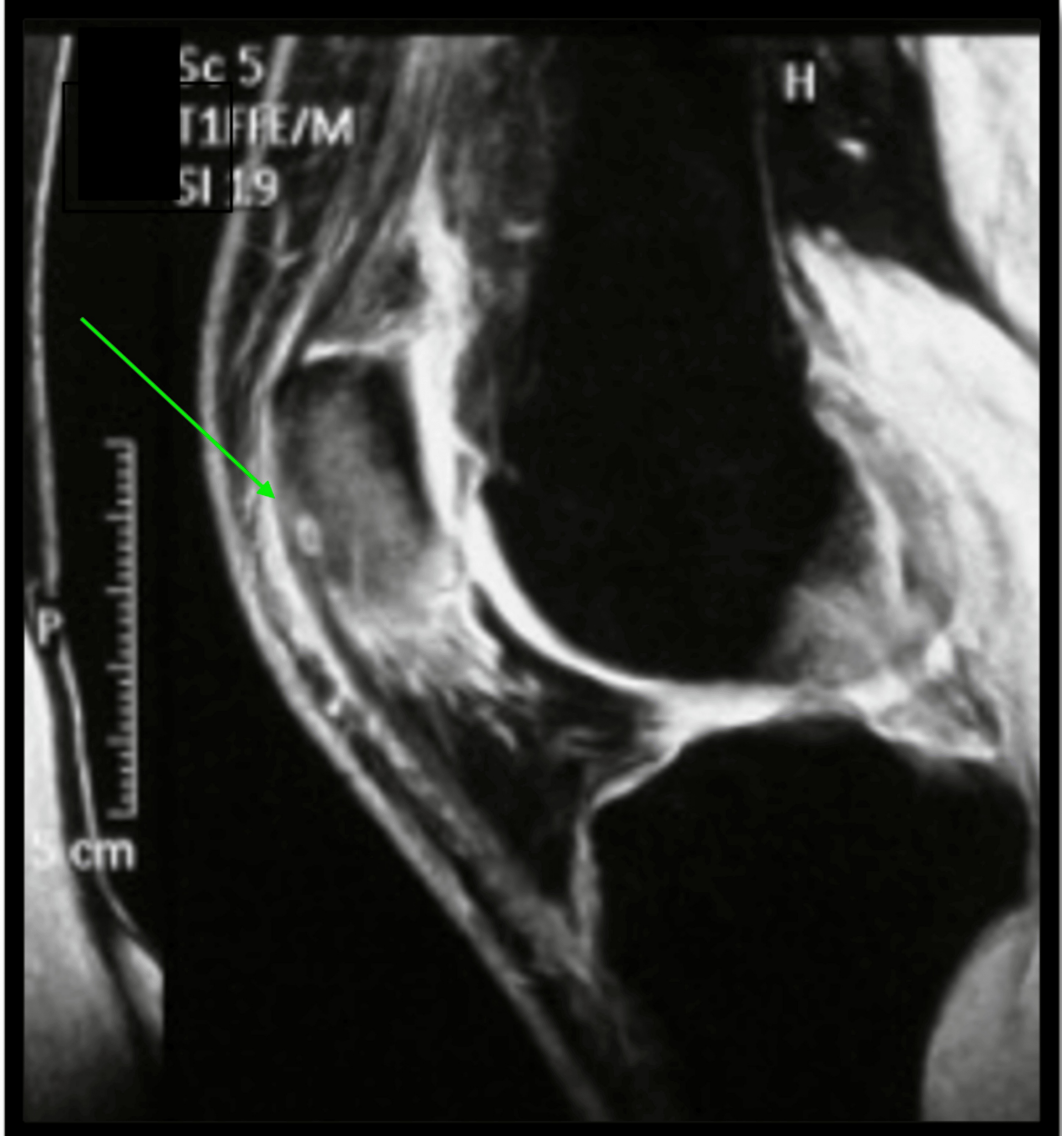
Sagittal view of T2-weighted magnetic resonance imaging (MRI) of the patella Diffuse bone marrow edema (high signal intensity) in the affected patella, and a well-circumscribed periosteal lesion at the inferior pole of the patella (green arrow).
